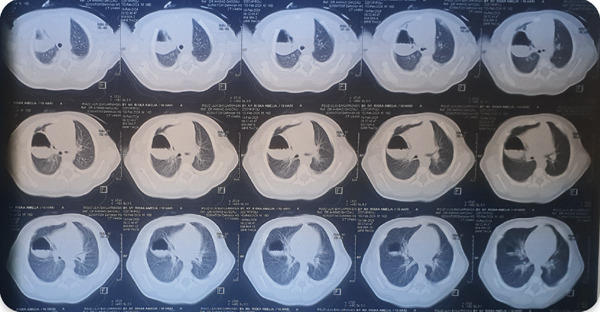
Pulmonary window of CT scan.
